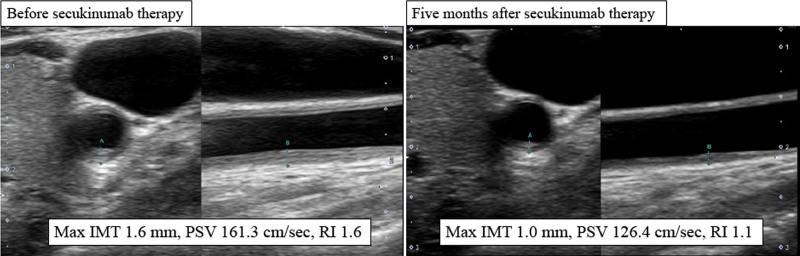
Changes in carotid artery ultrasound findings before and 5 months after secukinumab therapy. Five months after secukinumab treatment, ultrasound revealed improvement in the left common carotid artery wall thickening, as well as in blood flow velocity and vascular resistance. Left, transverse scan; right, longitudinal scan. IMT = intima-media thickness, PSV = peak systolic velocity, RI = resistance index.
